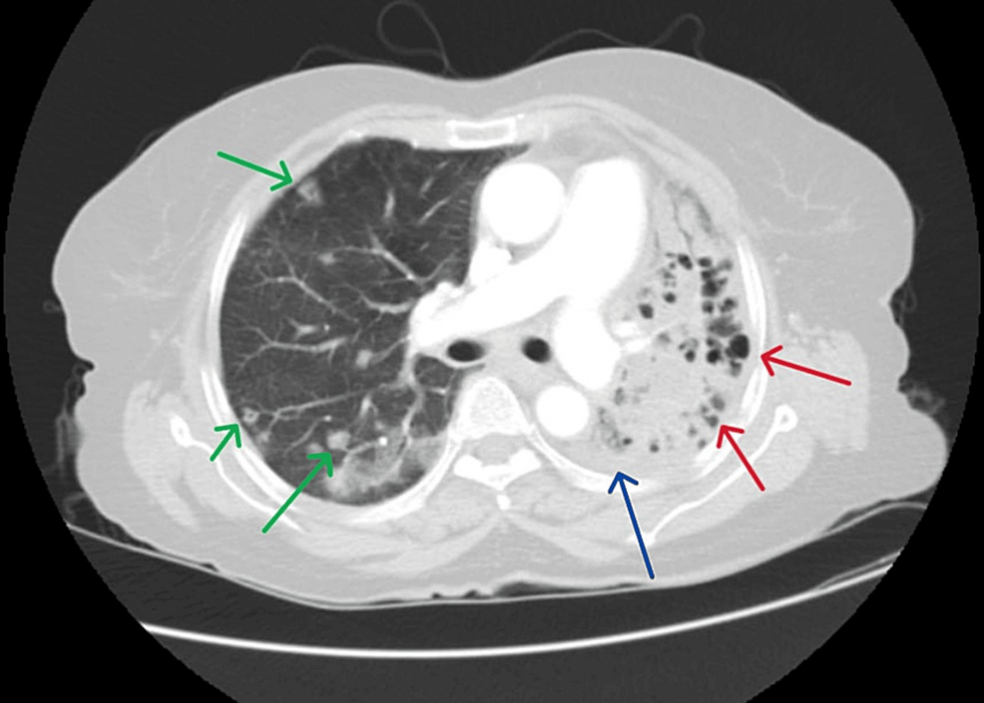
Contrast-enhanced computed tomography of the thorax showing multiple nodular lesions on the right side (green arrows) and cavitary lesions with dilated airways on the left (red arrows) with mild pleural effusion (blue arrow)
